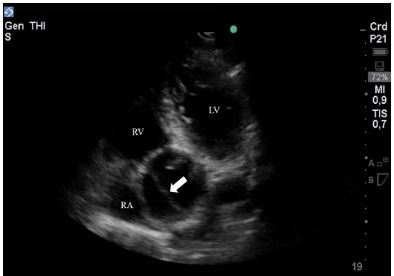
Transthoracic echocardiography apical 5-chamber view of a patient with acute chest pain with a dissection flap (white arrow) visualized in the ascending aorta. *LV*, Left ventricle; *RV*, right ventricle; *RA*, right atrium.
